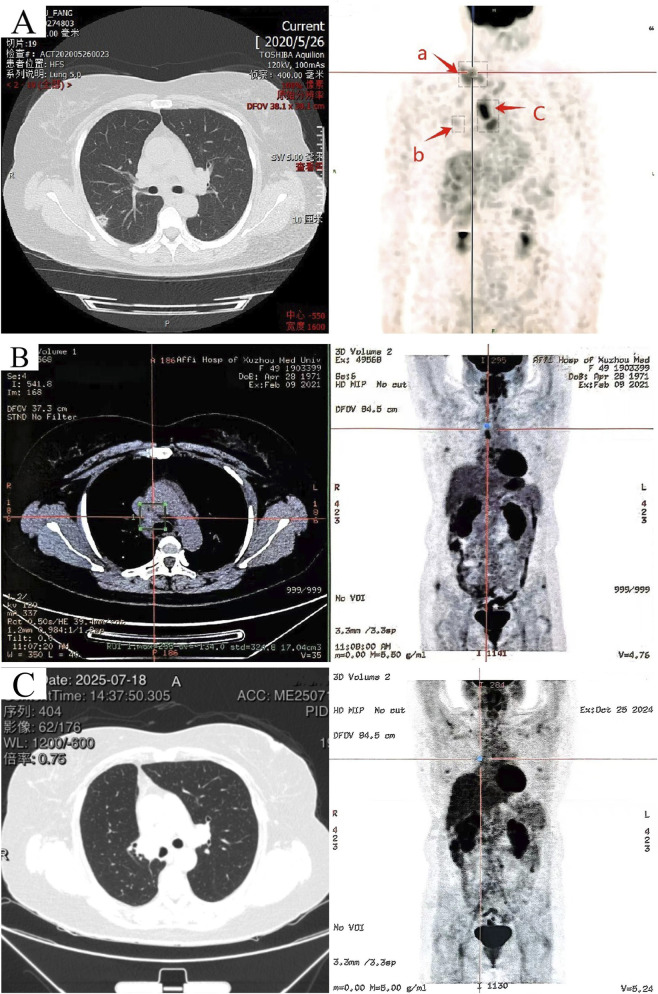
Imaging CT scans before crizotinib treatment, 8 months after crizotinib initiation, and 52 months postoperatively. (A) (June 2020) 64-row non-contrast chest CT and PET/SPECT-CT images: a nodular shadow in the right upper lobe (b), and focal abnormal elevation of glucose metabolism in multiple enlarged lymph nodes of the right supraclavicular fossa (a) and mediastinum (c). (B) (February 2021) PET-CT scan 8 months after crizotinib initiation: only hypermetabolic lymph nodes in the mediastinum. (C) Left: CT imaging from the follow-up in late July 2025. Right: PET-CT from October 2024 showing no increased glucose metabolism in mediastinal or clavicular lymph nodes.
